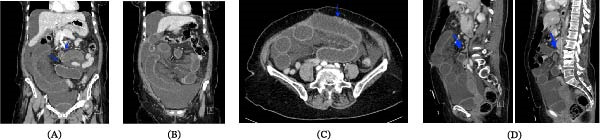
Computed tomography images. (A) Coronal image showing a dilated small bowel with two transition zones, suggesting a closed‐loop obstruction due to a transmesenteric internal hernia. (B) Coronal image of the abdomen showing dilated small bowel segments with mesenteric edema and fluid. A moderate ascites volume is also visible. (C) Axial image through the lower abdomen showing a dilated small bowel segment with mural thickening and decreased mural enhancement, suggesting bowel ischemia. (D) Sagittal images of the abdomen showing dilated small bowel segments with two transition zones (arrows) as well as mesenteric edema and free fluid.
